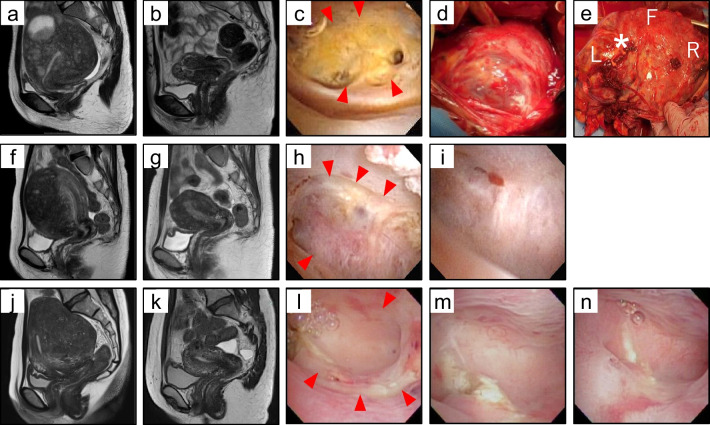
Endometrial defect and restoration after adenomyomectomy. (a, b) Case 1 magnetic resonance imaging (MRI) before and after adenomyomectomy. (c) Hysteroscopic finding in case 1. There was an endometrial defect at the uterine fundus (red arrowheads) 3 months after adenomyomectomy. (d) Intraoperative image of case 1 during cesarean section. The placenta is visible through the uterine serosa at the anterior wall of the uterus (white arrowheads). (e) Uterine rupture through the percreta observed at the posterior wall of the resected uterus. Multiple hemorrhages were observed through the vessels of percreta in the abdominal cavity during the cesarean section (*). “F,” “R,” and “L” indicate fundus, right, and left, respectively. (f, g) Case 4 MRI image before adenomyomectomy. (h, i) Hysteroscopic findings in case 4, endometrial defect at the uterine fundus surrounded by red arrowheads, and restoration 3 and 7 months after adenomyomectomy. (j, k) Case 5 MRI image before and after adenomyomectomy. (l, m, n) Hysteroscopic findings in case 5, endometrial defect at the uterine fundus surrounded by red arrowheads, and restoration 3, 6, and 8 months after adenomyomectomy
